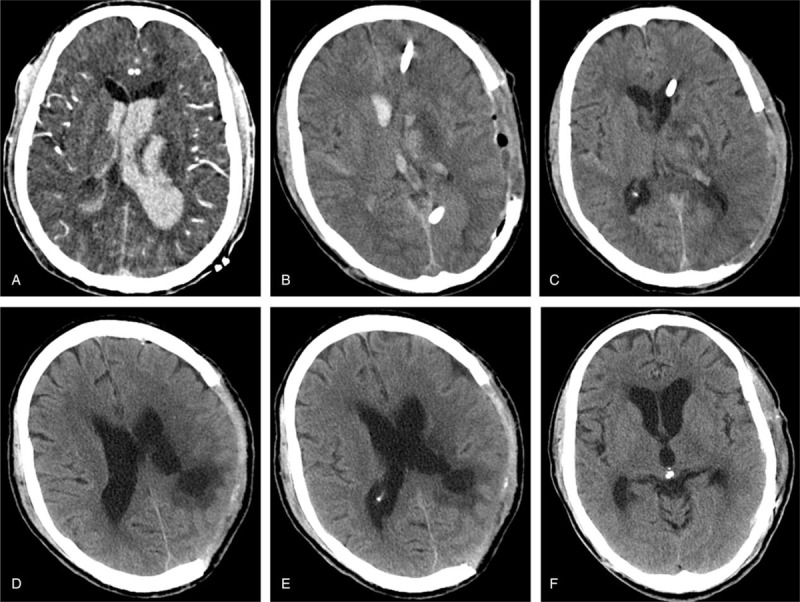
Imaging features of a representative case of paradoxical herniation. A, CT and Computed tomography angiography (CTA) upon admission, showing left occipital hematoma rupturing into the ventricular system and no vascular malformation. The GCS score was 9. A hematoma in the occipital lobe was removed. The decompressive craniectomy (left side) was followed by ventriculostomy. ICP returned to normal range after the surgery. B, CT scan postoperative day (POD) 1. C, CT scan on POD 5. At this time, the neurologic status deteriorated. ICP was 1 to 3 mm Hg. Systolic BP decreased to 75 mm Hg. External ventricular drainage was shut off, and the patient was placed in the Trendelenburg position with rapid intravenous hydration. D, CT scan on POD 14. CT = computerized tomography, GCS = Glasgow Coma Scale, ICP = intracranial pressure.
